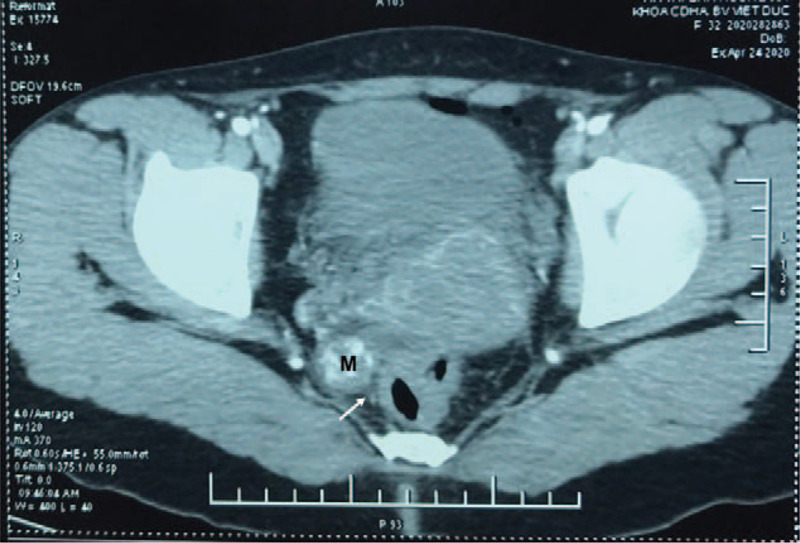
Abdominal CT angiography on the day of admission (M: mass, arrow: mass in contact with the rectal wall).
